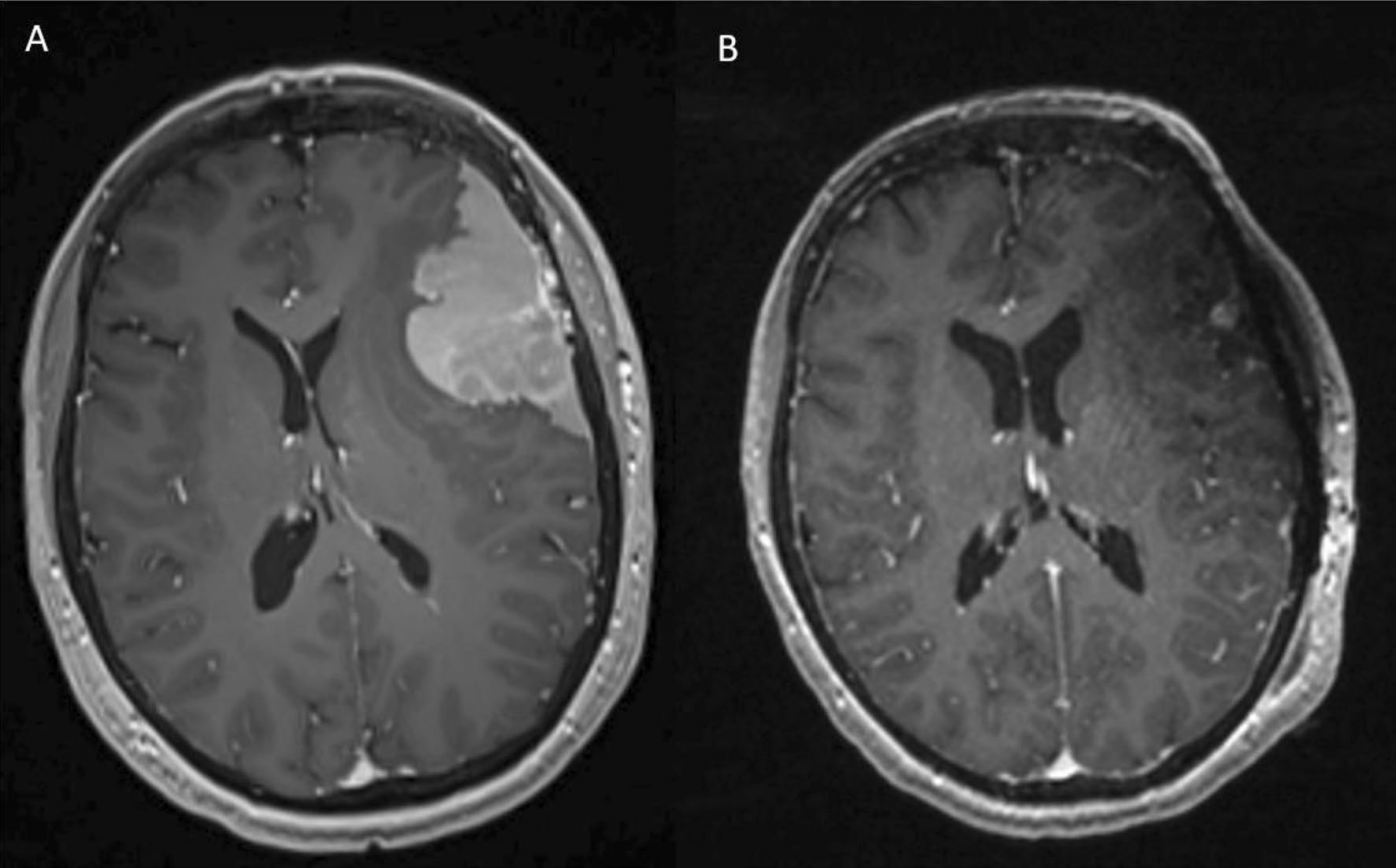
A 60-year-old right-handed female presented with an episode of word-finding difficulty. Axial MRI with contrast demonstrates an enhancing extra-axial lesion in the left frontotemporal convexity causing mass effect and surrounding edema (A). There is concern for bone invasion, which was confirmed intraoperatively. The patient underwent a Simpson grade I resection and mesh cranioplasty (B). Pathology confirmed a grade 1 meningioma with NF2 mutation
